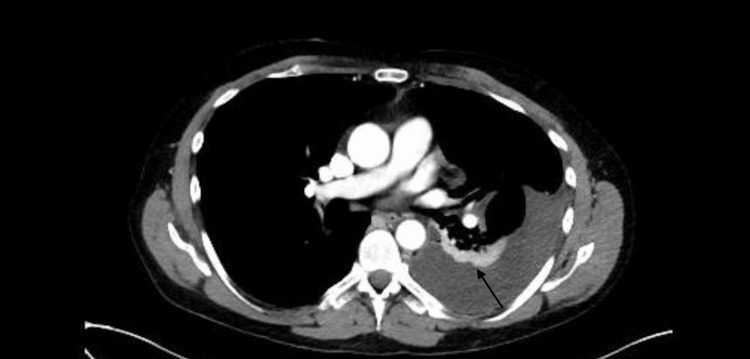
Chest computed tomography demonstrating a large left pleural effusion The arrow indicates a hematocrit sign. There are two different densities in the collection because cellular components in effusion settle to a dependant region. The Hounsfield units were compatible with subacute blood products. The Hounsfield units were 49 HU.
